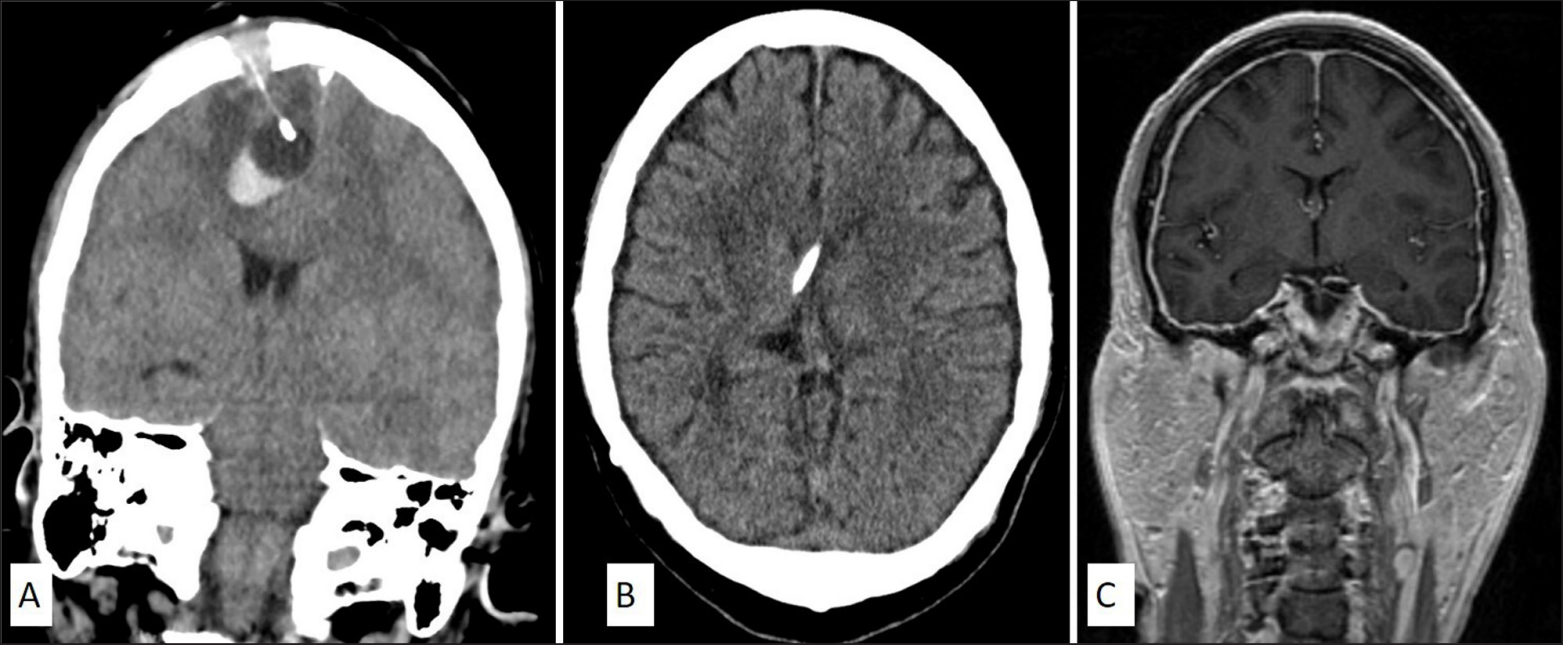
Coronal CT image of a 57-year-old man with severe brain injury who underwent insertion of a right frontal intracranial pressure (ICP) monitoring probe (A) demonstrates an acute intraparenchymal hematoma at the tip of the ICP monitoring probe. Axial CT brain of a 54-year-old man (B) shows ventricular catheter of a right parietal ventriculoperitoneal shunt abutting the septum pellucidum. The ventricles are slit-like. Coronal post-contrast T1-Weighted MRI of the man (C) confirms slit-like ventricles along with pachymeningeal thickening and enhancement. This is consistent with intracranial hypotension due to over-drainage of CSF.
